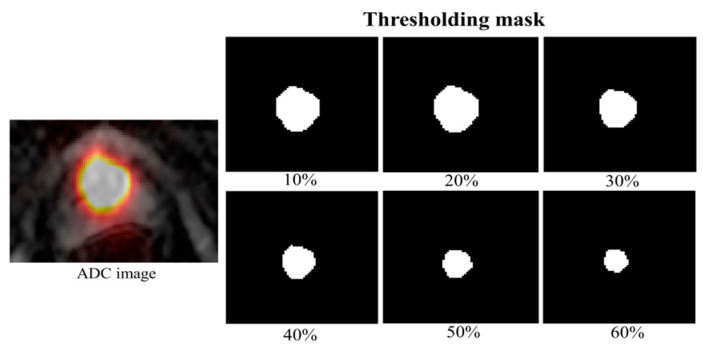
ADC map overlaid over the PET image of the prostate cancer colored in Hot (Left column). Six different ROI masks for cancer were created by applying a percentage threshold of SUV_max_ values of 10%, 20%, 30%, 40%, 50%, and 60% (Right column).
